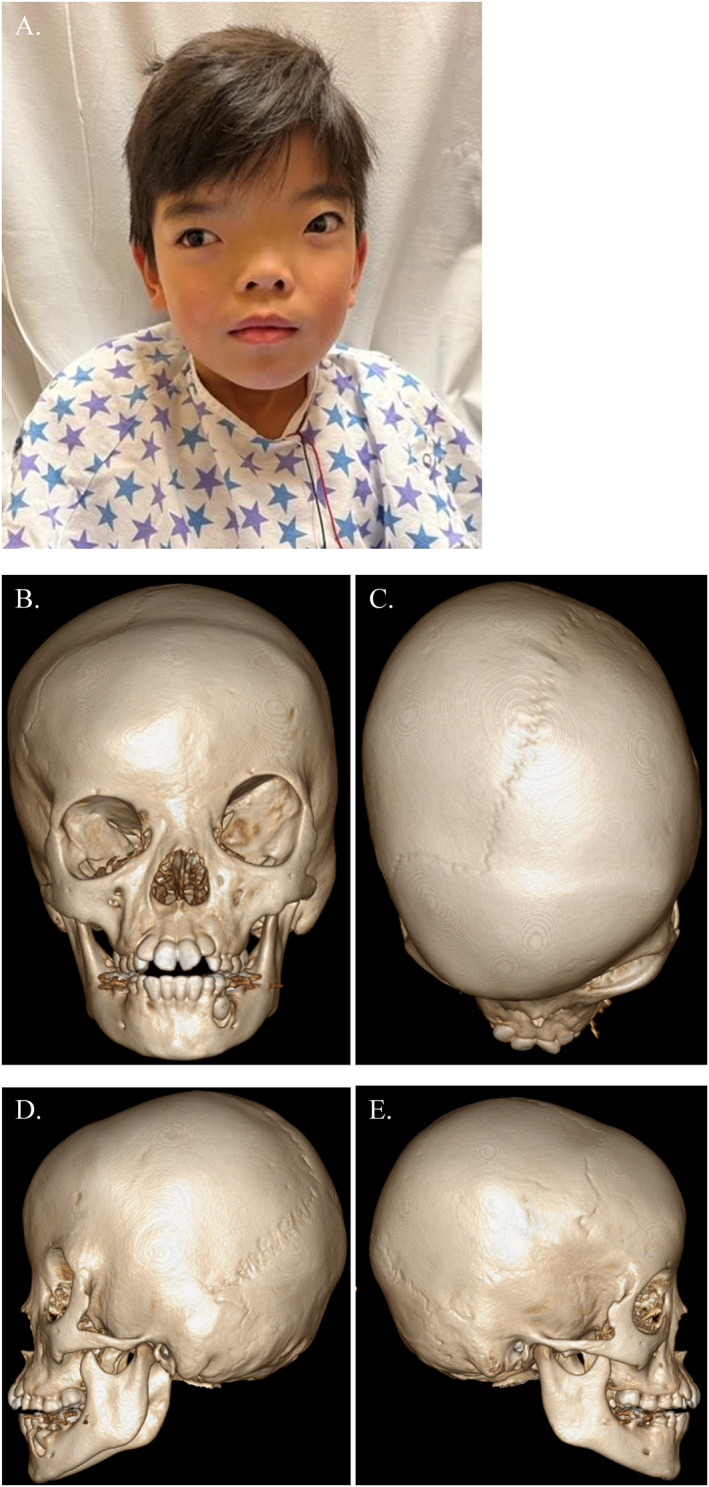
(A) Pre‐operative patient photo. (B–E) Pre‐operative three‐dimensional CT imaging.
